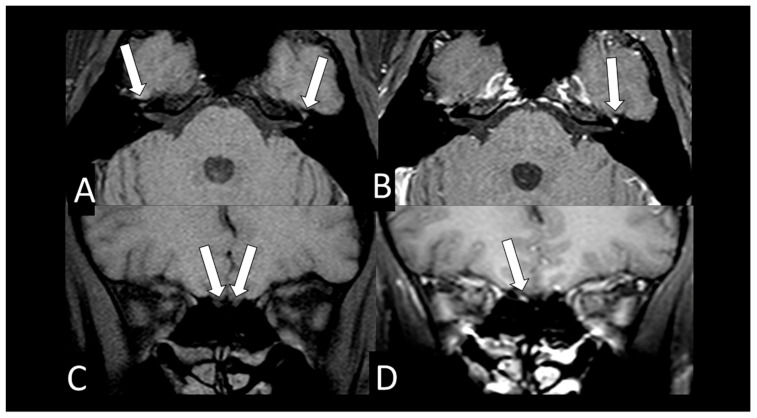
Case 32—The MRI shows both hypointense geniculate ganglions on axial fat suppressed T1 WI (A, arrows), but the left geniculate ganglion has abnormal stronger enhancement on axial post contrast (B, arrow) fat suppressed T1 WI. The coronal fat suppressed T1 WI shows olfactory bulbs with normal hypointense signal (C, arrows), but the post contrast coronal fat suppressed T1WI shows abnormal enhancement in the right olfactory bulb (D, arrow).
